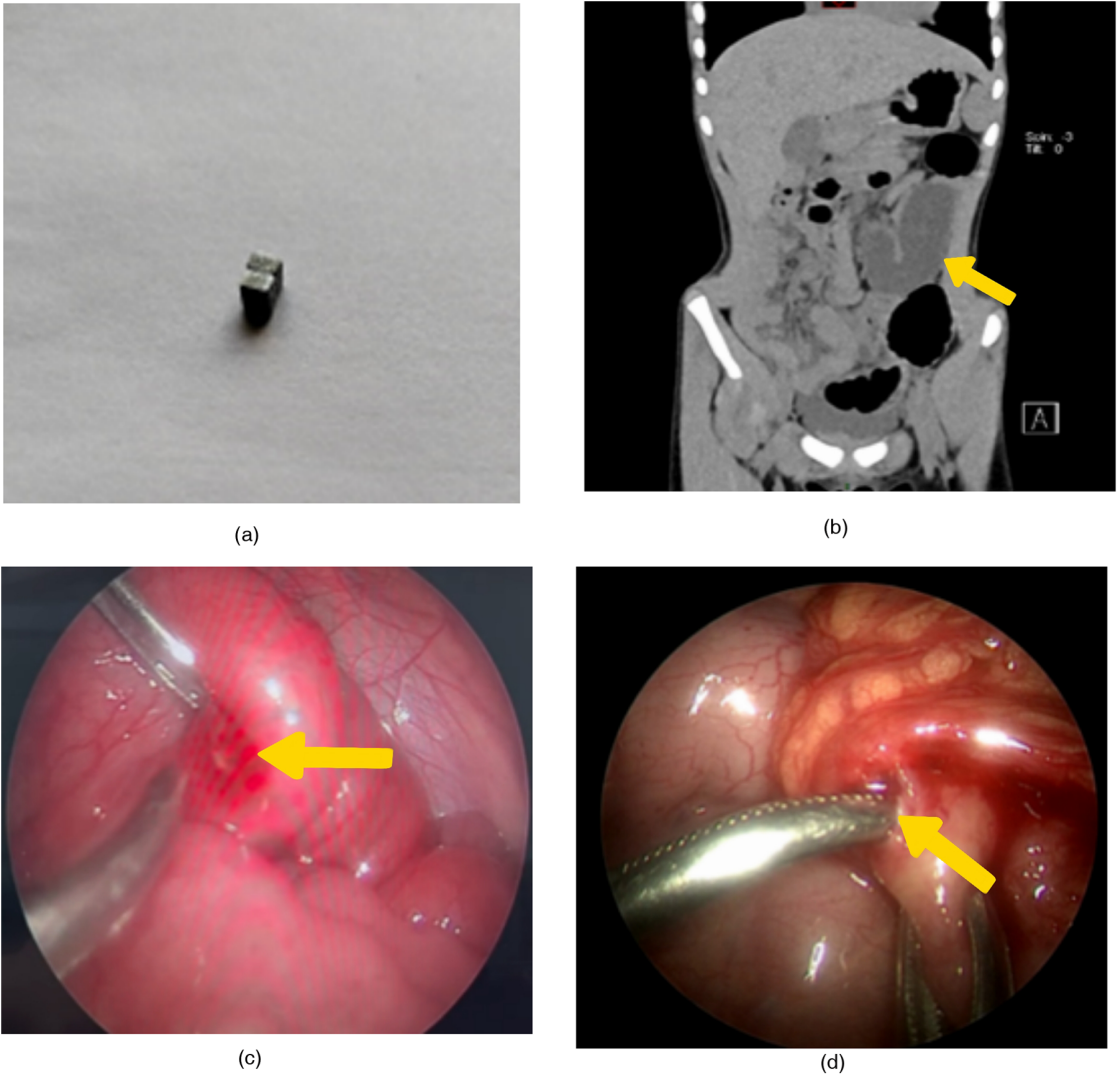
(a) the mother provided two homotypic square magnets, which were identical to the magnet the child had been playing with but not swallowed. (b) The non-contrast CT scan shows marked dilation of the proximal small intestine and complete emptiness of the distal bowel. Intestinal obstruction is considered. (c) Enteric perforation located 60 cm from the ileocecal valve in the ileum. (d) Colonic perforation located in the mid-transverse colon.
